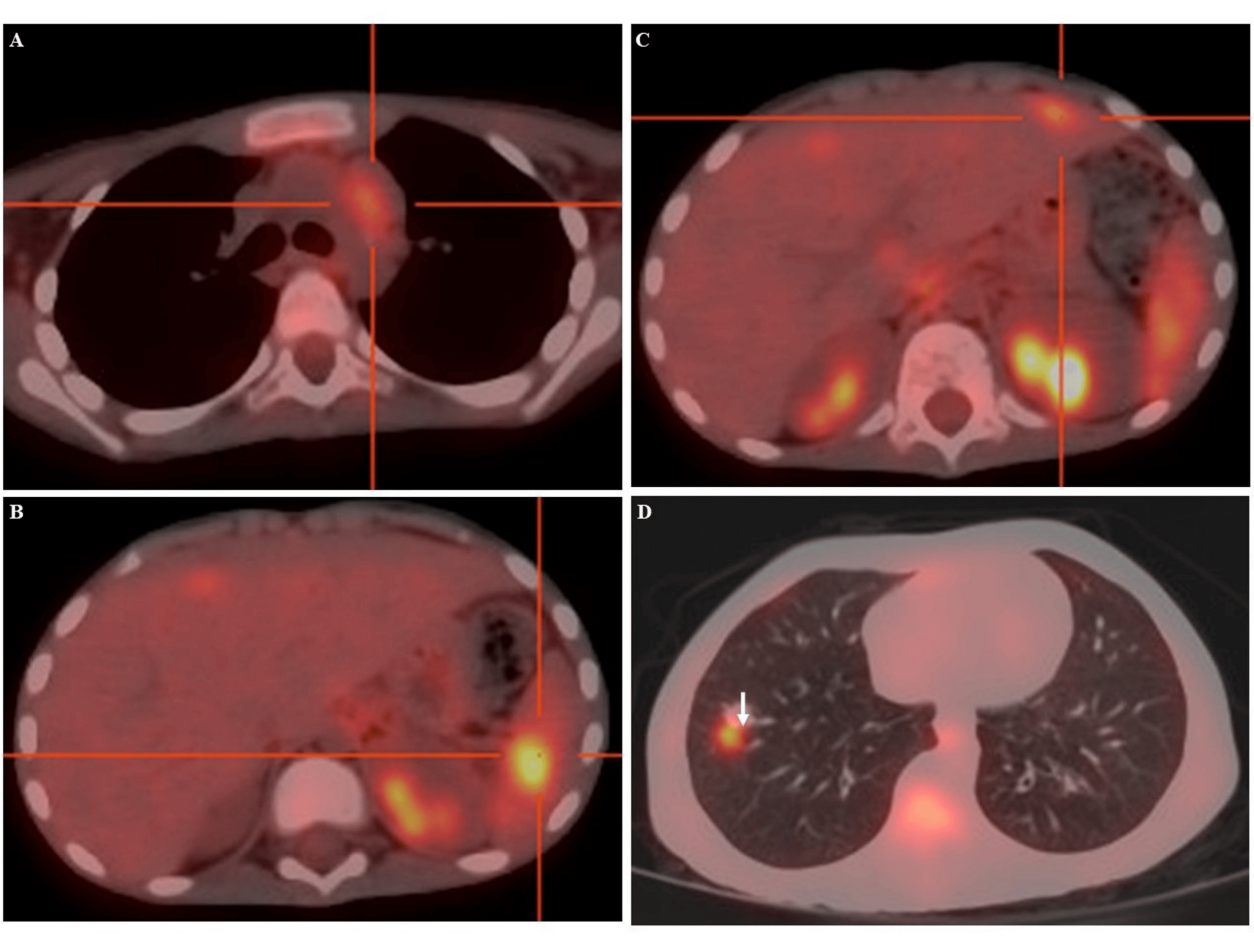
PET-CT axial views of hypermetabolic lesions on prevascular node (A), spleen (B) and liver (C) nodes, as well as a pulmonary nodule (D).
